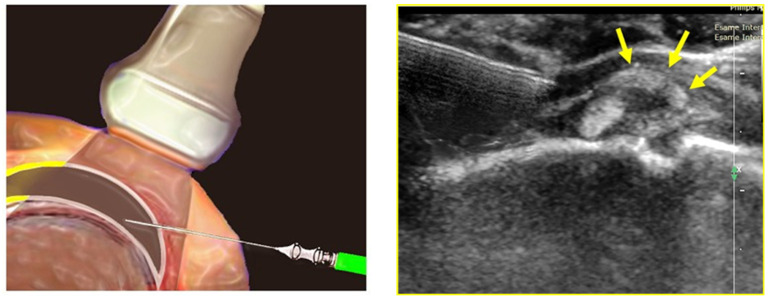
Ultrasound-guided infiltrative treatment.
